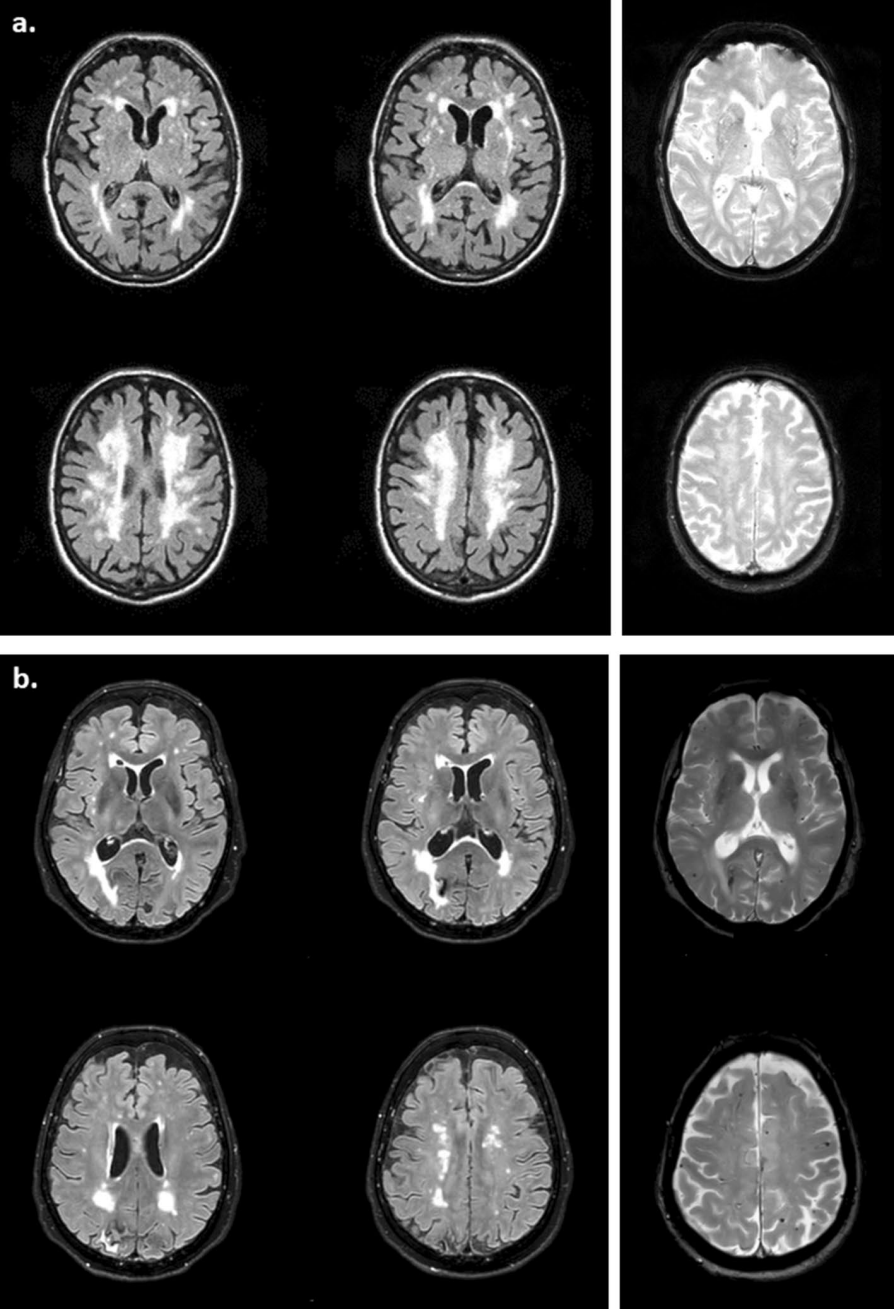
Examples of representative brain of HA and CAA patients. (a) Example of a HA patient: Man, 88 years old. History of lacunar stroke three years before; MCI diagnosis according to neuropsychological evaluation. His Brain MRI shows severe subcortical and periventricular WMH (Fazekas 3) in FLAIR sequences. T2* shows one right deep thalamic CMB, with no evidence of lobar CMBs. (b) Example of a CAA patient: Woman, 78 years old. History of lobar ICH two years before (right occipital); CAA diagnosis according to modified Boston criteria. Her Brain MRI shows multiple subcortical WMH spots (Fazekas 2) in FLAIR sequences. T2* shows several lobar CMBs, with no evidence of deep CMBs.
